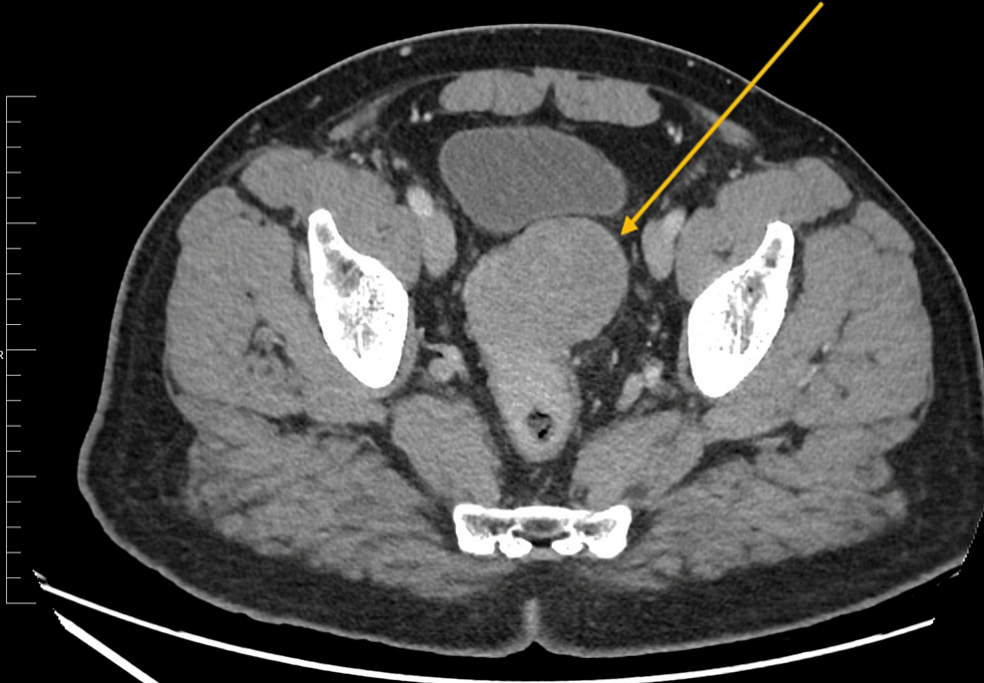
Axial abdominal CT scan showing a well-defined mass with heterogeneous enhancement arising from the third part of the rectum.
